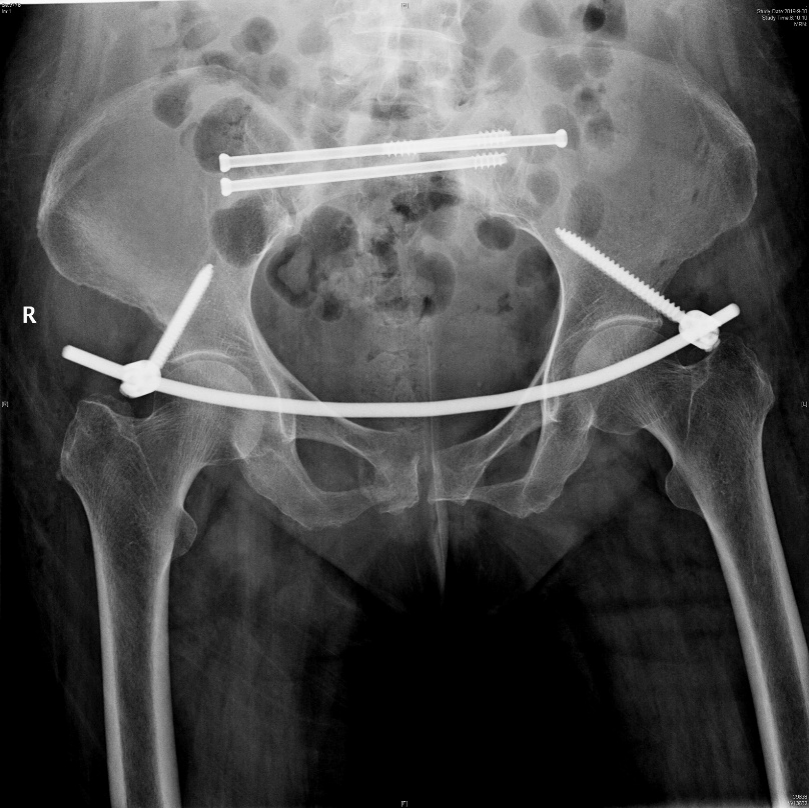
X-ray postoperation showed anterior pelvic ring internal fixator for symphysis pubis fracture combined iliosacral screws for sacrum fracture.
